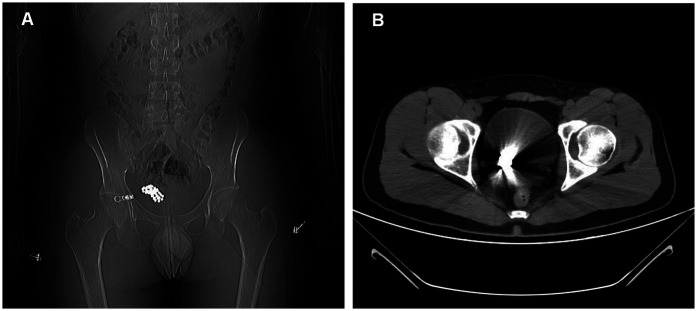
Pelvic plain computed tomography (CT) image. (A) Pelvic plain CT localizer radiograph showing numerous metal beads. (B) Plain pelvic CT scan being affected by metal artifacts.
